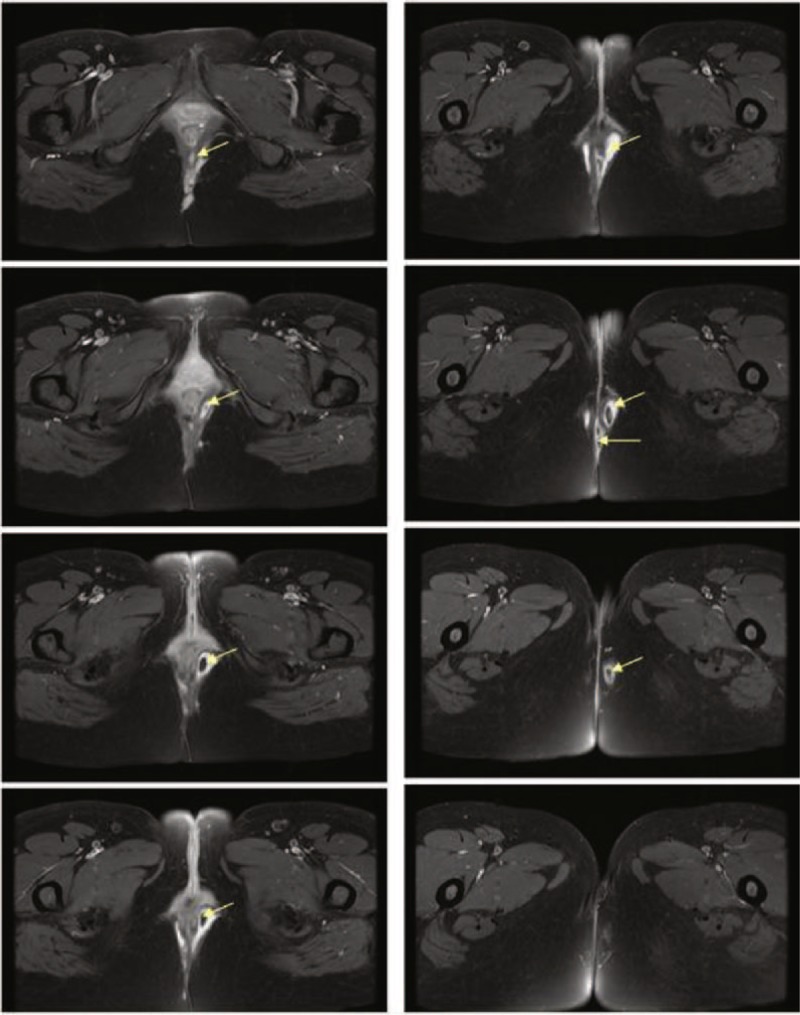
MRI of the patient's pelvic cavity revealed small multifocal areas (arrows) on the left, right, and back side of the anus. These foci had a weaker intensity on the T1WIs and were more heterogeneous with stronger intensity on the T2-weighted images. Note that the left side of the pelvic cavity (L) appears larger than the right side (R). MRI = magnetic resonance imaging, T1WI = T1-weighted image.
